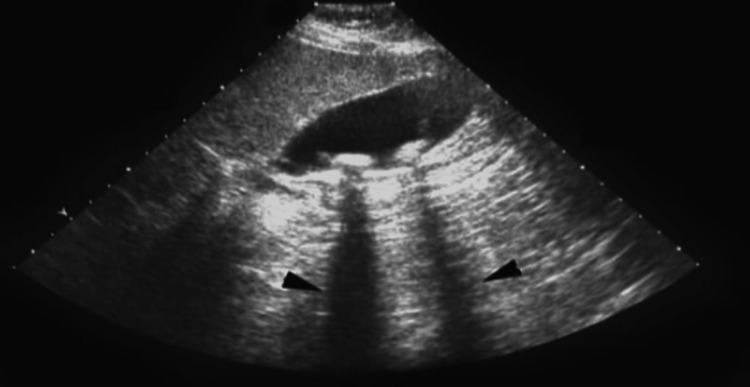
Ultrasound of the gallbladder. Arrows indicate posterior acoustic shadowing from gallstones.
